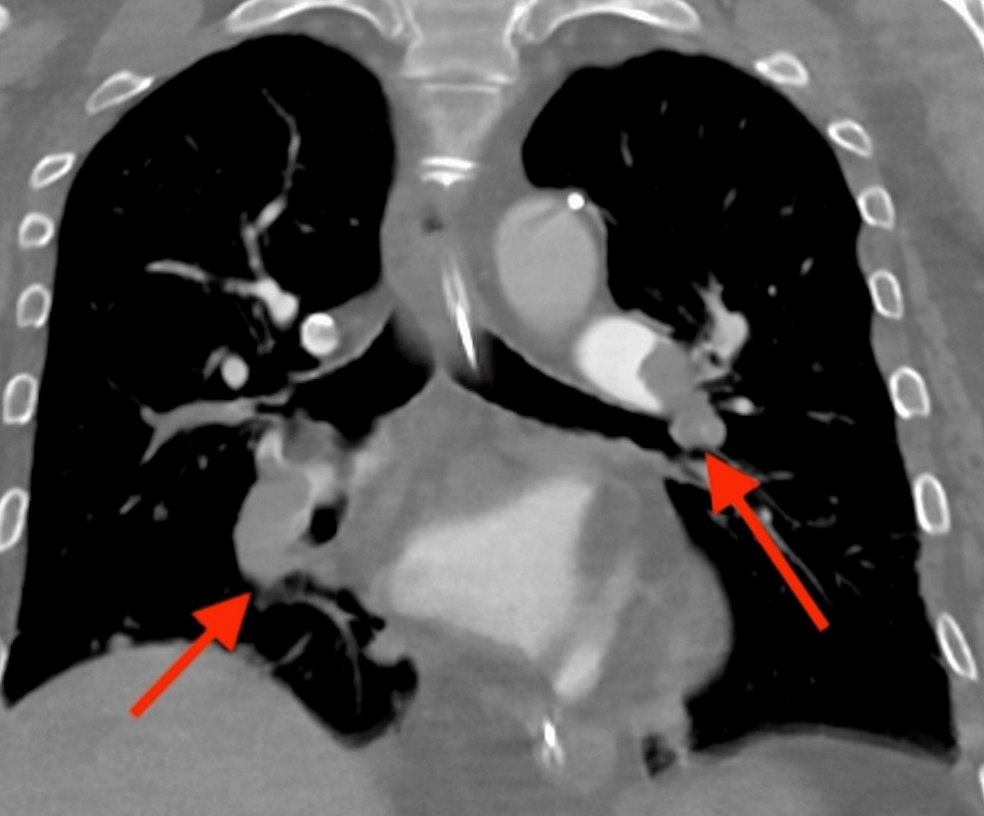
A CT pulmonary angiogram upon initial presentation demonstrates left main pulmonary arterial and right lower lobar thromboembolism (red arrows) CT: computed tomography
